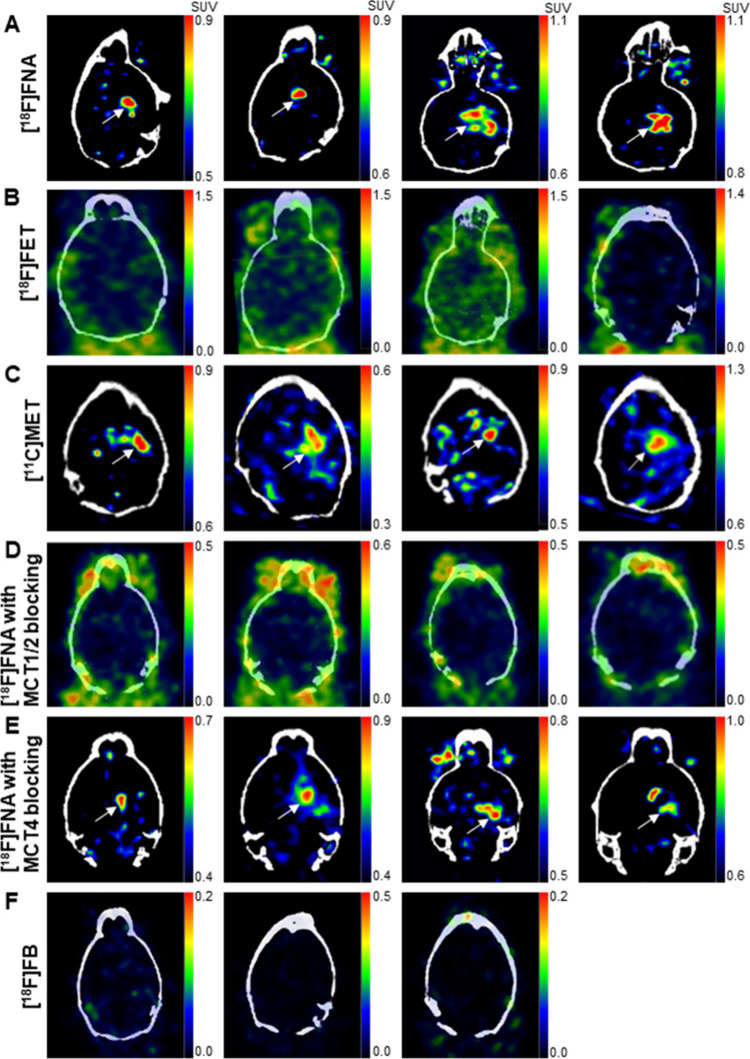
Time-weighted mean PET/CT images of intracranial glioblastoma xenograft
